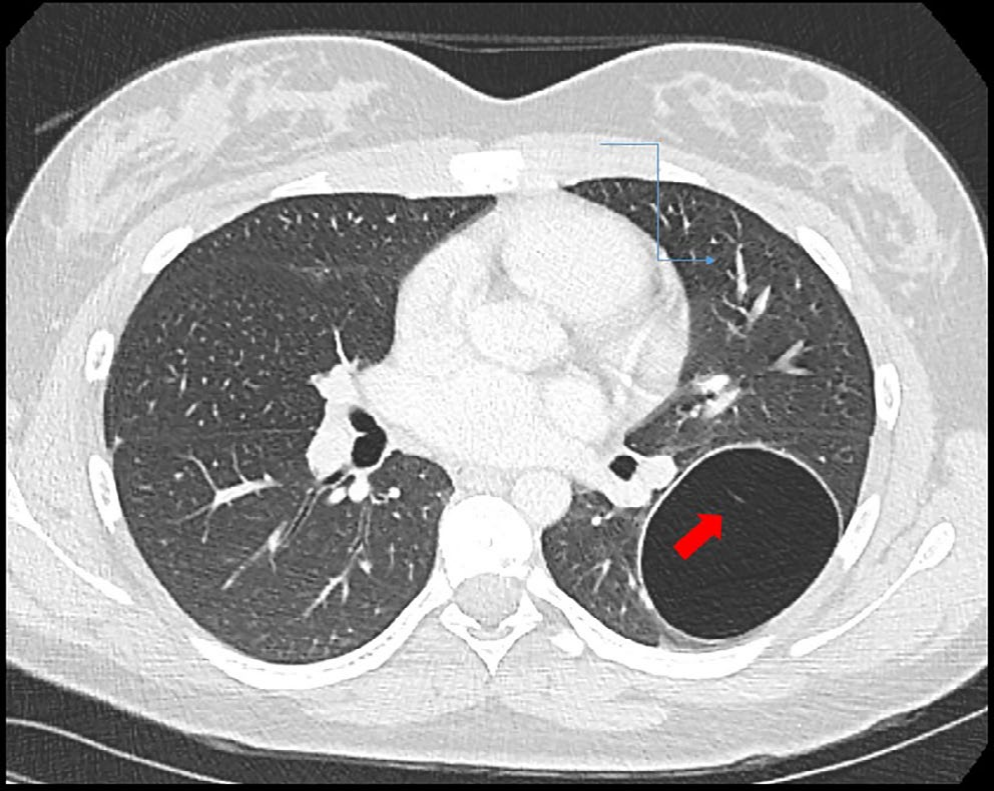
Axial lung window CT image shows an air‐filled cystic lesion within the left lower lobe. The lesion demonstrates a slightly thick wall and a subtle internal linear septation (arrow)
